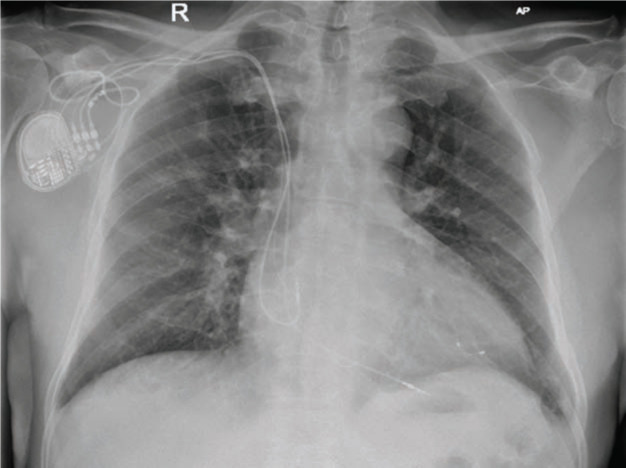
Chest radiograph of the repositioning of the right ventricular pacing lead into the right ventricular apex.
